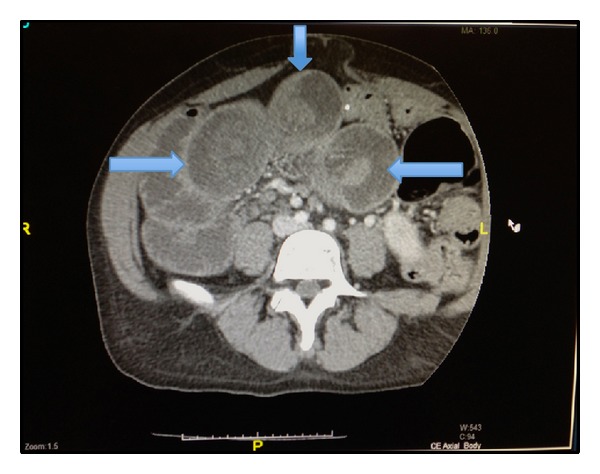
Abdominal CT scan with target signing of bowel (arrows).
